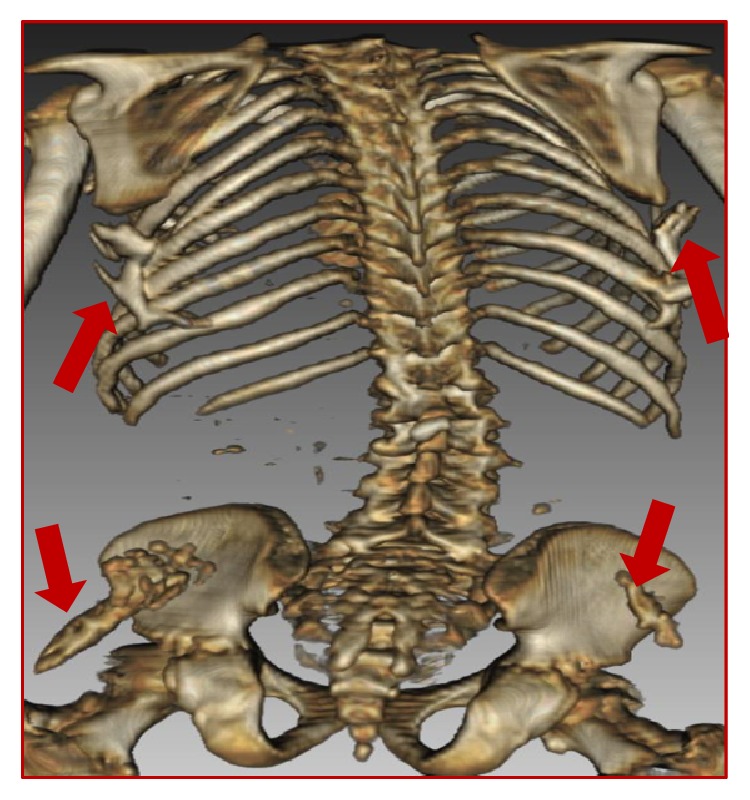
Three-dimensional reconstruction CT: presence of extraskeletal ossifications of the posterior surface of the trunk and the posterior surface of the iliac wings (red arrows).
